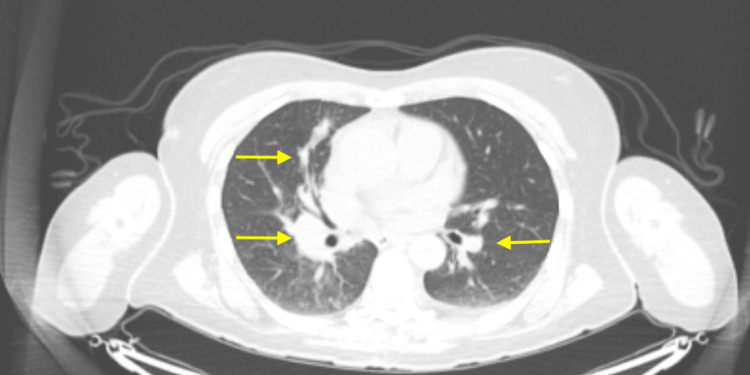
August 2023 CT chest showing response to radiation and immunotherapy. Yellow arrows point to the same mass-like consolidations from Figure 1, which are decreased in size.
